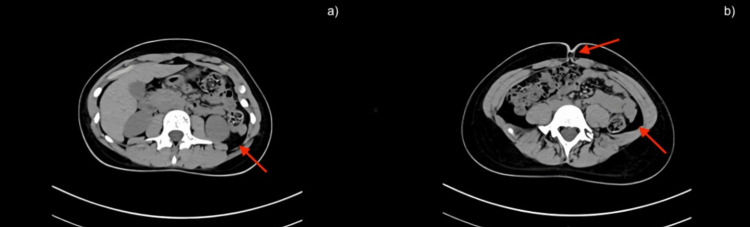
Axial abdominal CT scan. (a, b) Free fluid is observed in the posterior cul-de-sac. Small umbilical hernia. Findings consistent with mesenteric adenitis. CT: computed tomography
